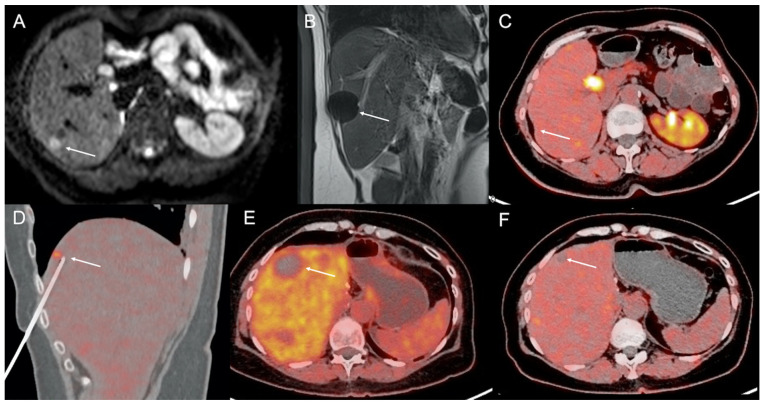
A 55-year-old woman with grade 1 ileal NEN liver metastases and a previous history of MRI-guided liver metastasis ablation. MRI diffusion-weighted axial image showed a target liver metastasis (arrow) of segment VI (A), effectively treated with percutaneous MRI-guided cryoablation (B, arrow: iceball), which resulted in a complete retraction of the ablation site without any pathologic ^18^F-DOPA uptake during follow-up PET/CT (C, arrow). Four years after the first treatment, the patient underwent ^18^F-DOPA PET/CT-guided radiofrequency ablation of a 10 mm liver metastasis (D, arrow) of the IV liver segment. Immediate postablation ^18^F-DOPA PET-CT showed complete tumor destruction without residual ^18^F-DOPA uptake (E, arrow). ^18^F-DOPA PET/CT performed 24 months later showed limited parenchymal scarring without pathologic ^18^F-DOPA uptake (F, arrow).
